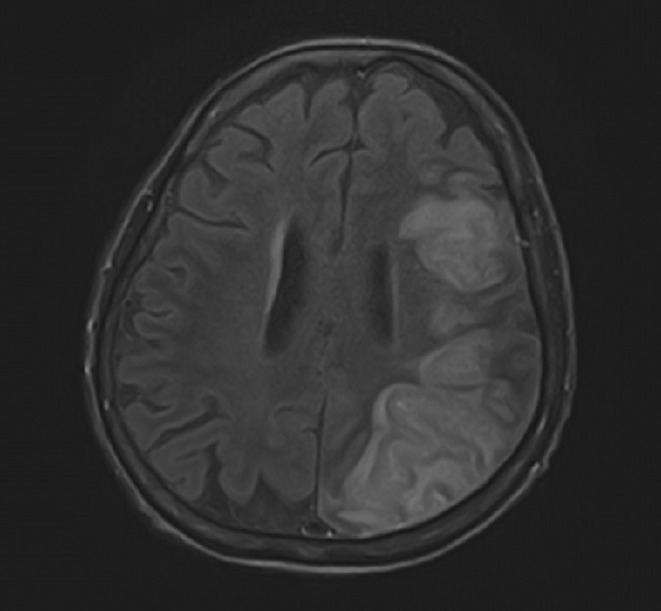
FIGURE 1 MRI axial FLAIR image
